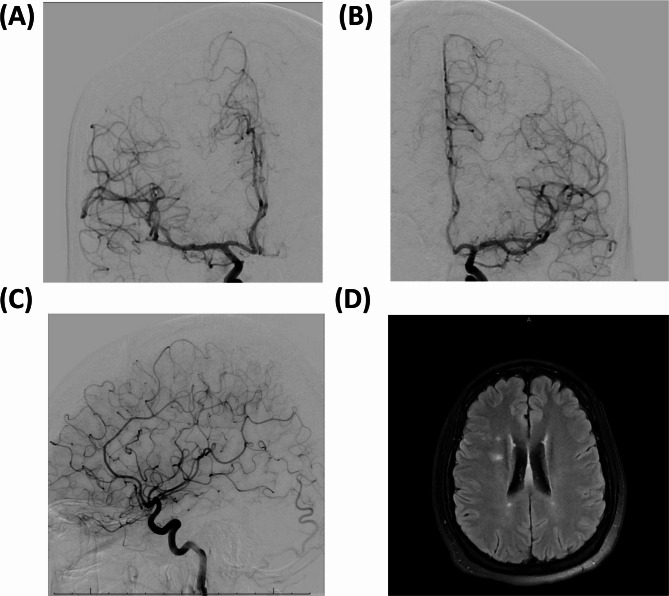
Right ICA AP injection showing focal and multifocal segmental narrowing of both A3 and A4 segments of ACA and M3 and M4 segments of MCA (A), Left ICA AP injection also showing decreased caliber of distal branches of both ACA and MCA(B), Left ICA Lateral injection again showing decreased caliber of distal branches of both ACA and MCA (C), MRI T2 flair showing hyperintensities representing presumed vasculitis related infarcts in bilateral hemispheres (D)
